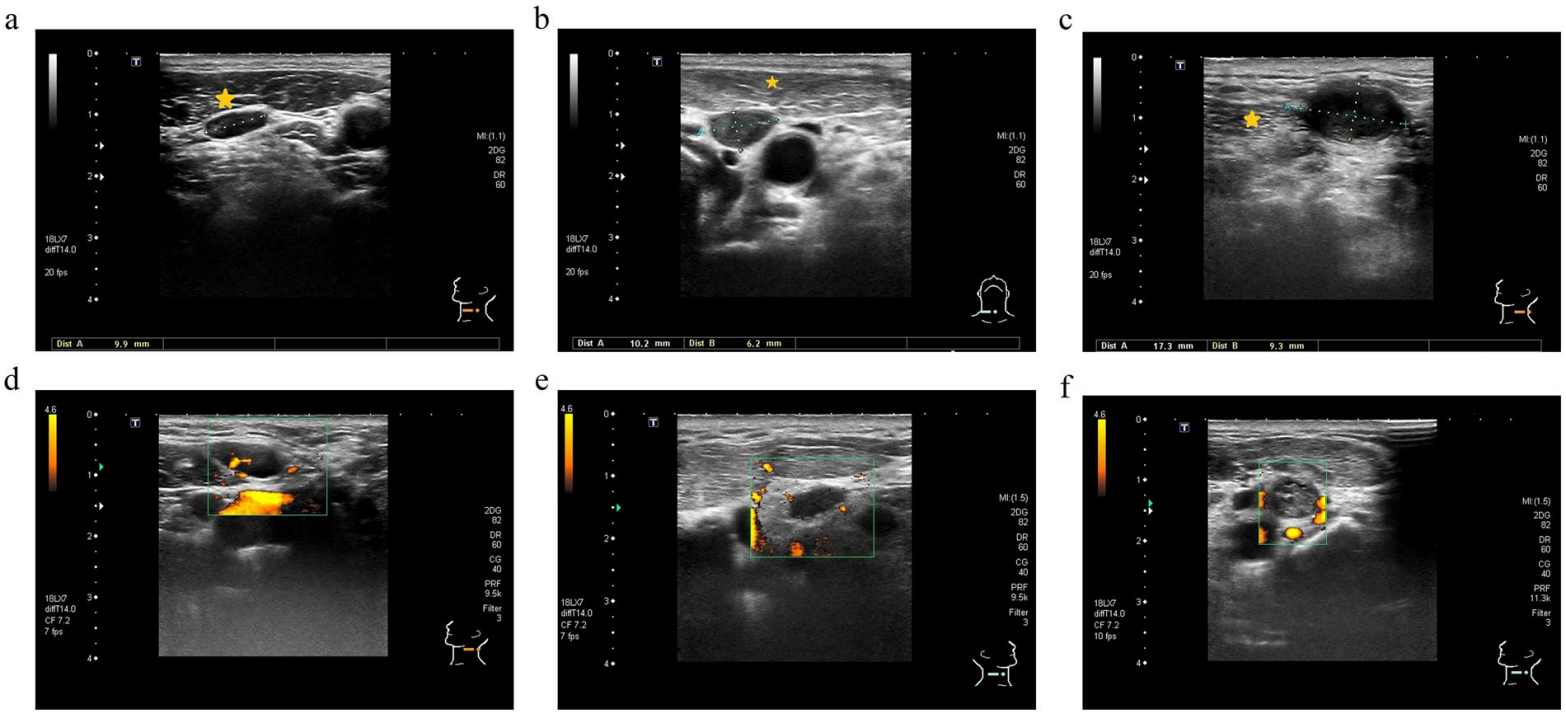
In comparison to the untreated neck, there are two ultrasound features of lymph nodes (hypoechogenicity and avascular pattern) that are more frequently noted in the treated neck: (a) Left level III benign LN in the untreated neck, isoechogenicity (equal to left sternocleidomastoid muscle (star) without fibrotic change) (b) Right level IV non-recurrent LN in the treated neck, hypoechogenicity (lower than right sternocleidomastoid muscle (star) with fibrotic change after previous neck dissection) (c) Left level Va recurrent LN in the treated neck, hypoechogenicity (lower than left sternocleidomastoid muscle (star) with fibrotic change after radiation) (d) Left level IIa benign LN in the untreated neck with typical linear hilum using a Doppler scan (e) Right level III non-recurrent LN in the treated neck, avascular pattern using a Doppler scan (f) Left level III recurrent LN in the treated neck, avascular pattern using a Doppler scan.
